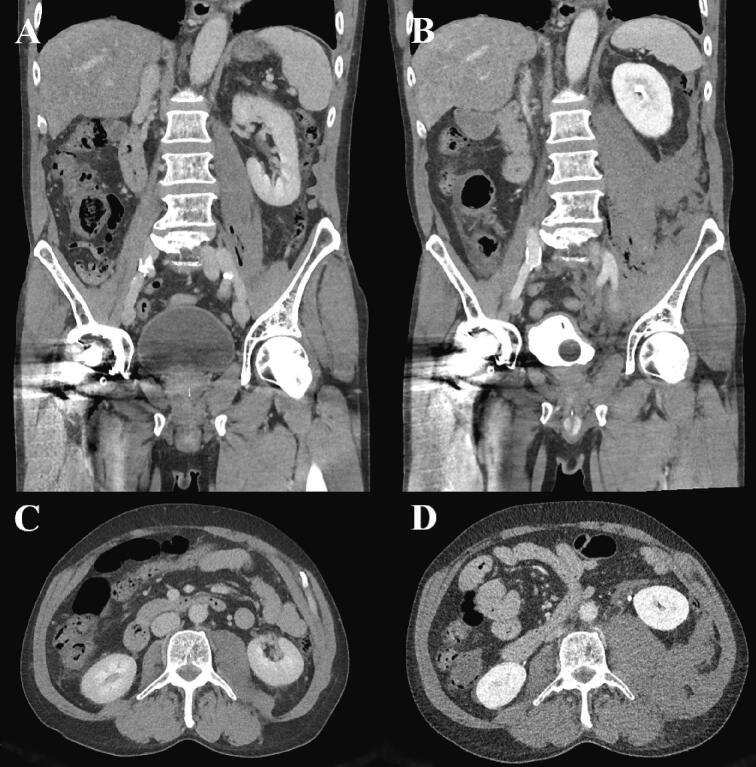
Abdominal CT on day 1. (A) Coronal and (C) axial planes from CT on admission display edema around the left iliopsoas muscle and minor gas formation within the muscle compartment. (B) Coronal and (D) axial planes from CT 2.5 h after admission display progression of the edema and gas formation. The left kidney is displaced anteriorly due to the collection of fluid, attenuating as blood, around the left iliopsoas muscle.
